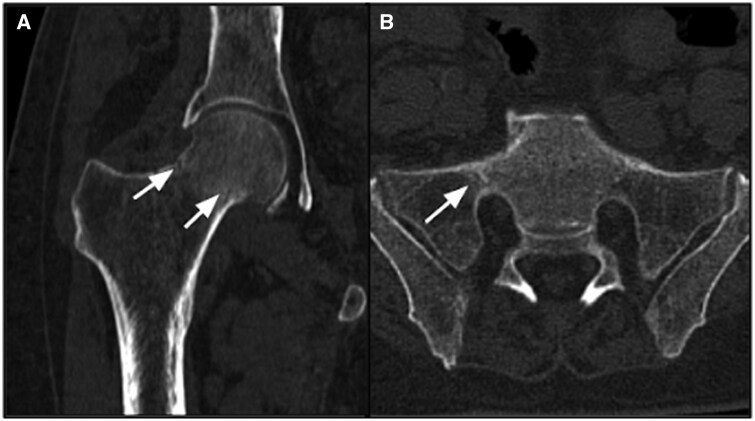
CT scan of pelvis shows insufficiency fractures in right femoral neck and ala of sacrum—fracture line in neck of right femur (white arrows) on coronal plane image (A). Thin fracture line is seen in the right ala of sacrum (white arrow) on axial plane image (B).
